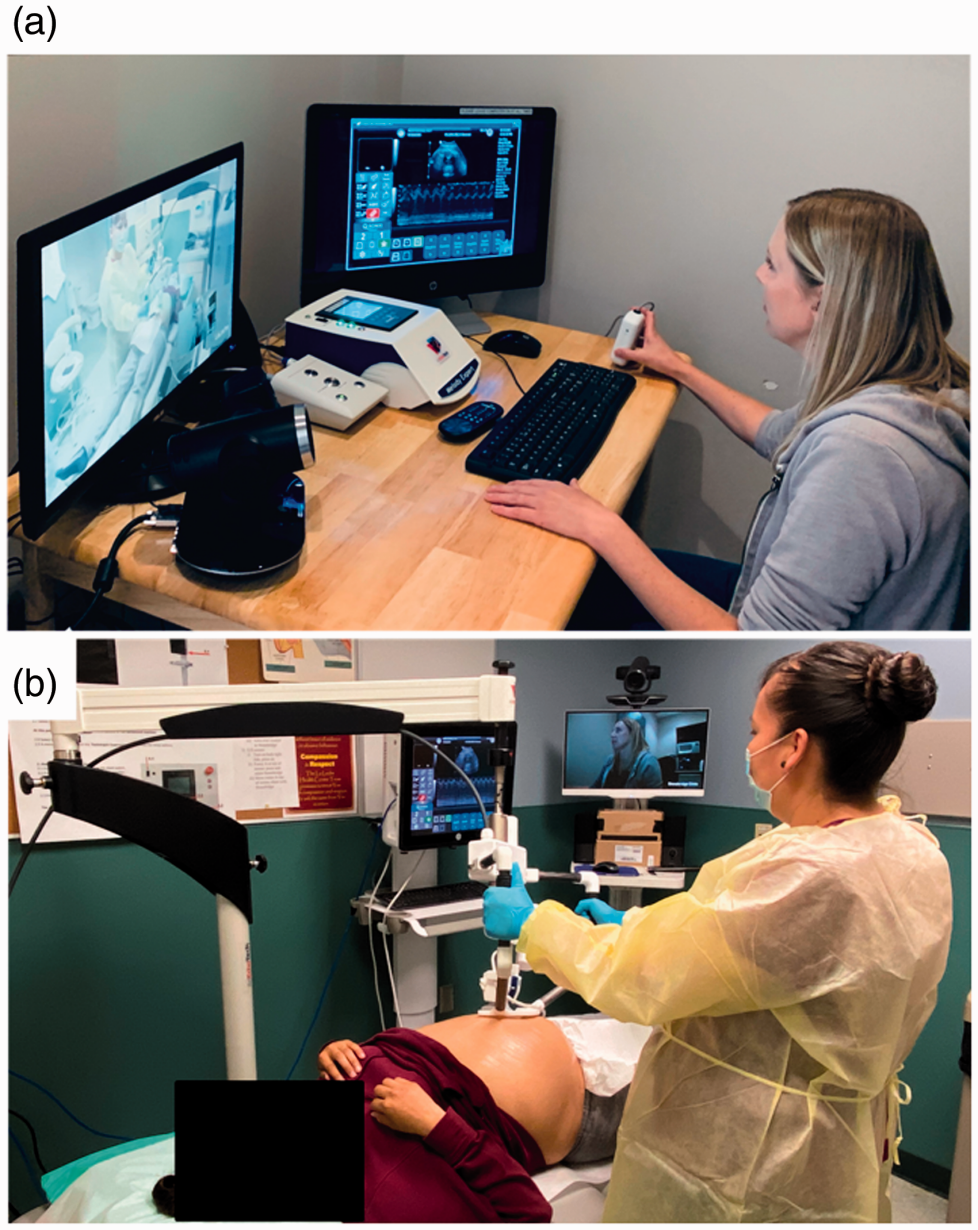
Telerobotic ultrasound system used during the coronavirus disease 2019
